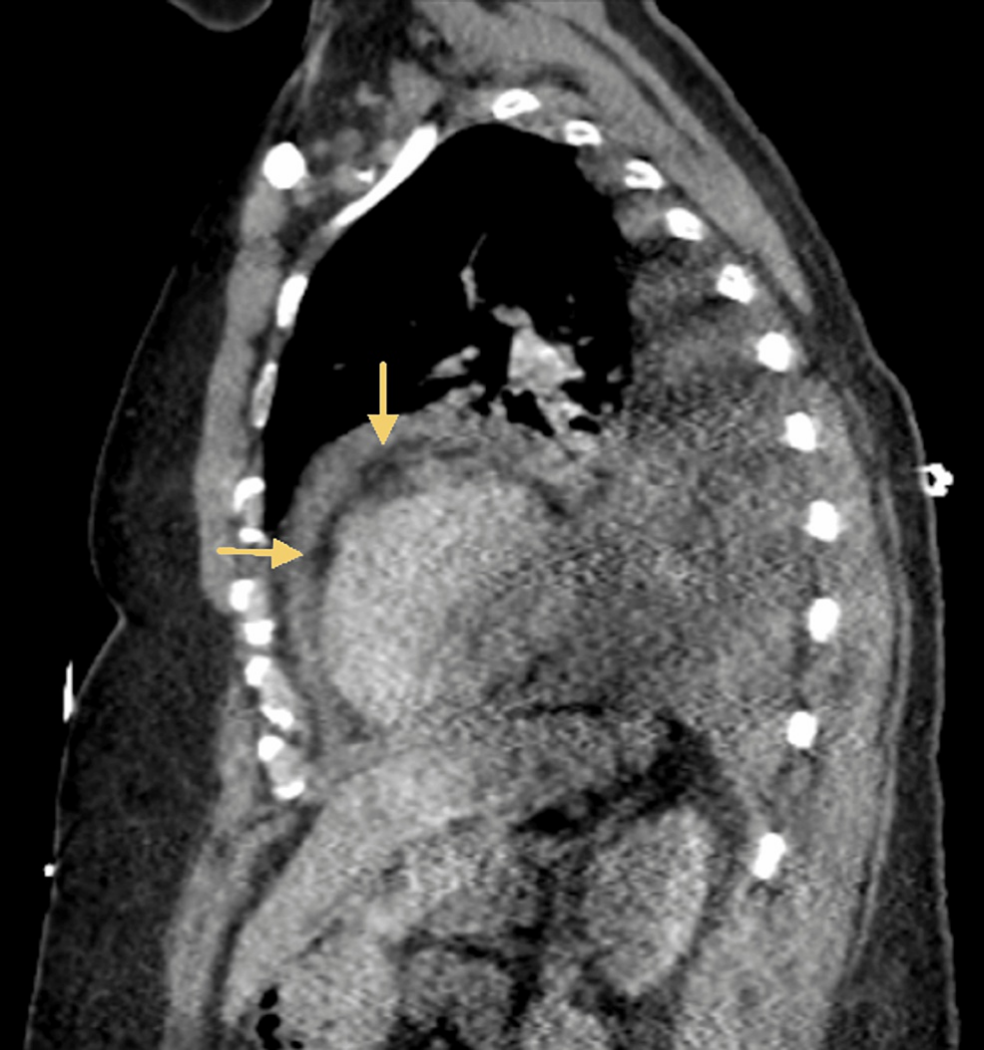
Computed tomography sagittal view of thoracic cavity. Note small pericardial effusion surrounding the myocardium (arrows).
